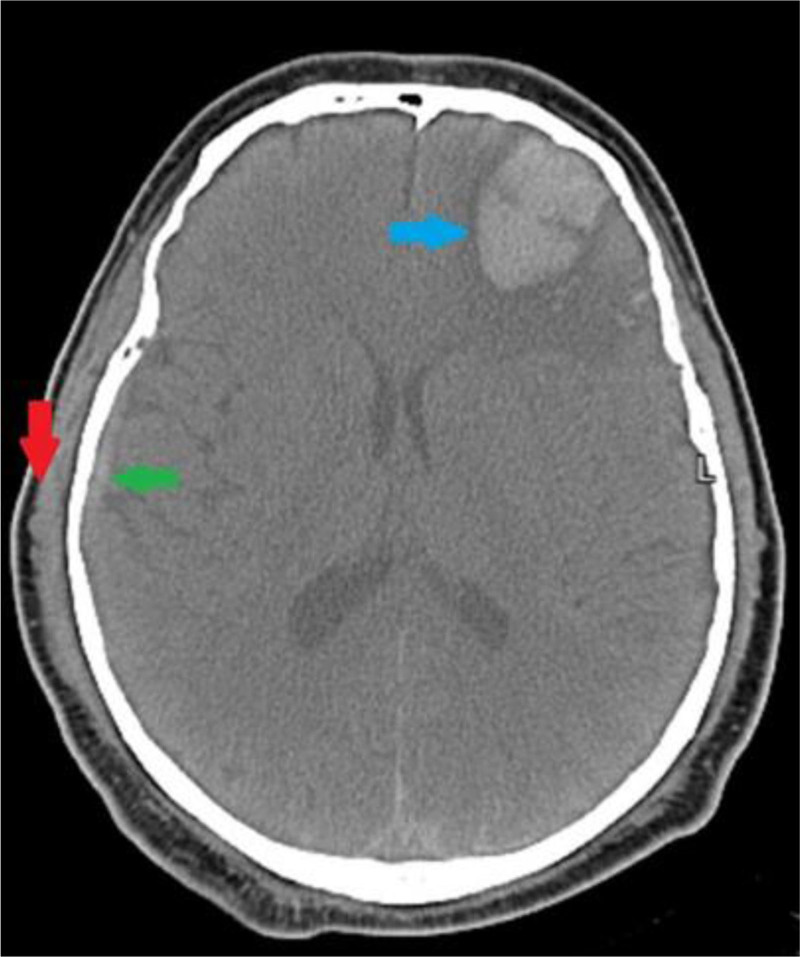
A follow-up non-contrast head CT for the same patient, demonstrating significant interval improvement. The right parietal epidural hematoma has markedly decreased in size, with associated resolution of the pneumocephalus (green arrow). The linear skull fracture remains visible but without evidence of complication. The bilateral subarachnoid hemorrhage is less conspicuous, and the left tentorial subdural hematoma (blue arrow) shows no progression in size. Importantly, there is no evidence of midline shift or significant mass effect. CT = computed tomography.
